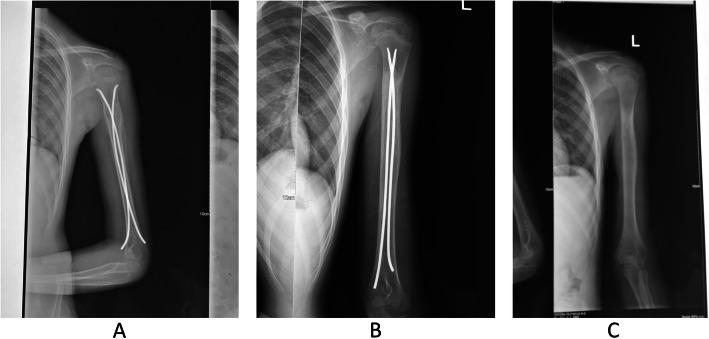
Mixed bone grafting fusion was indicated by imaging CT and X-ray during the follow-up period
